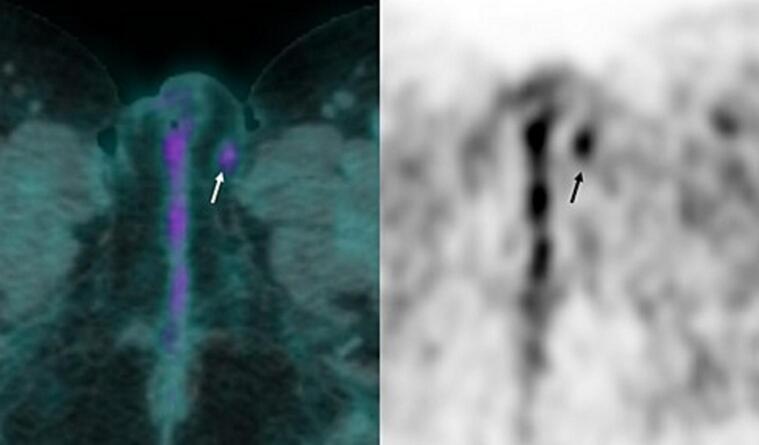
^18^F-FDG PET/CT of tumor recurrence. The PET-CT shows a moderate ^18^F-FDG uptake of the recurrence, with a SUV_max_ value of 2,9 g/mL, compatible with a neoplasm.
